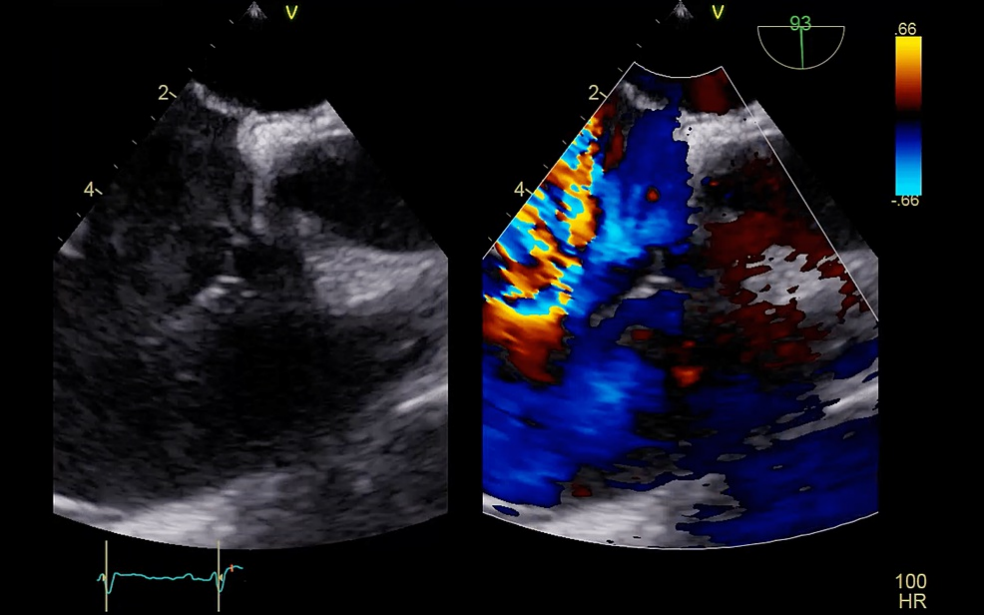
A modified bicaval view with color comparison of the last transesophageal echocardiogram showing a flail anterior TV leaflet with severe TR and no vegetation.
